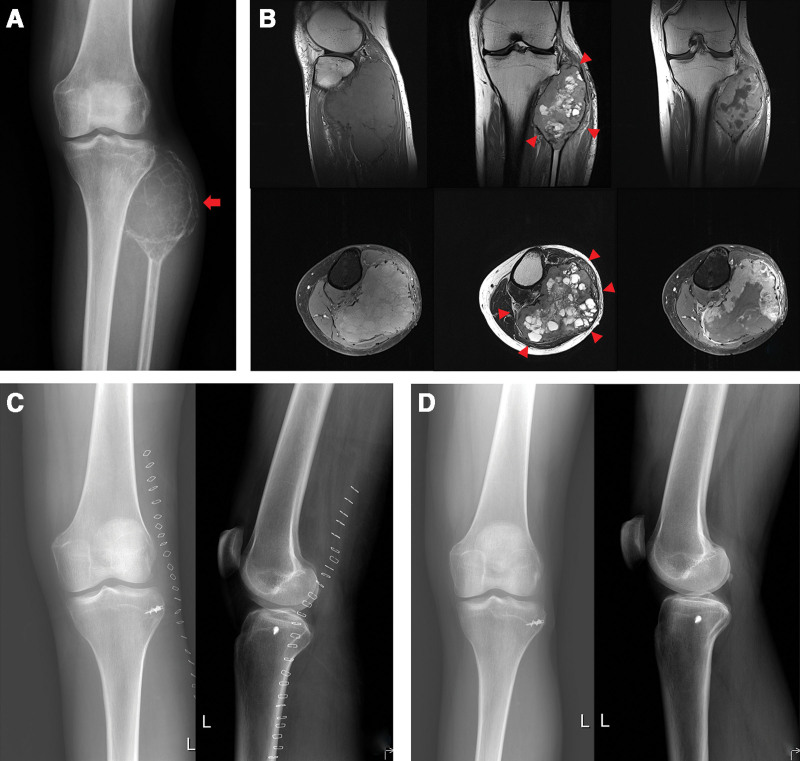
A female (patient 2 in Tables 1 and 2), who was 37 years old at diagnosis of giant cell tumor of bone (GCTB) in the left proximal fibula, reported no symptoms before pregnancy. However, after becoming pregnant, she noticed a rapid enlargement of a lump near her knee. (A) An anteroposterior plain radiograph of both knees showed an expansile bone lesion (an arrow). (B) Coronal and axial magnetic resonance images demonstrated GCTB with extraosseous extension (arrowheads). (C) Radical resection and re-attachment of the lateral collateral ligament to the proximal tibia were performed. (D) No local recurrence was detected 4 years after surgery. GCTB = giant cell tumor of bone.
